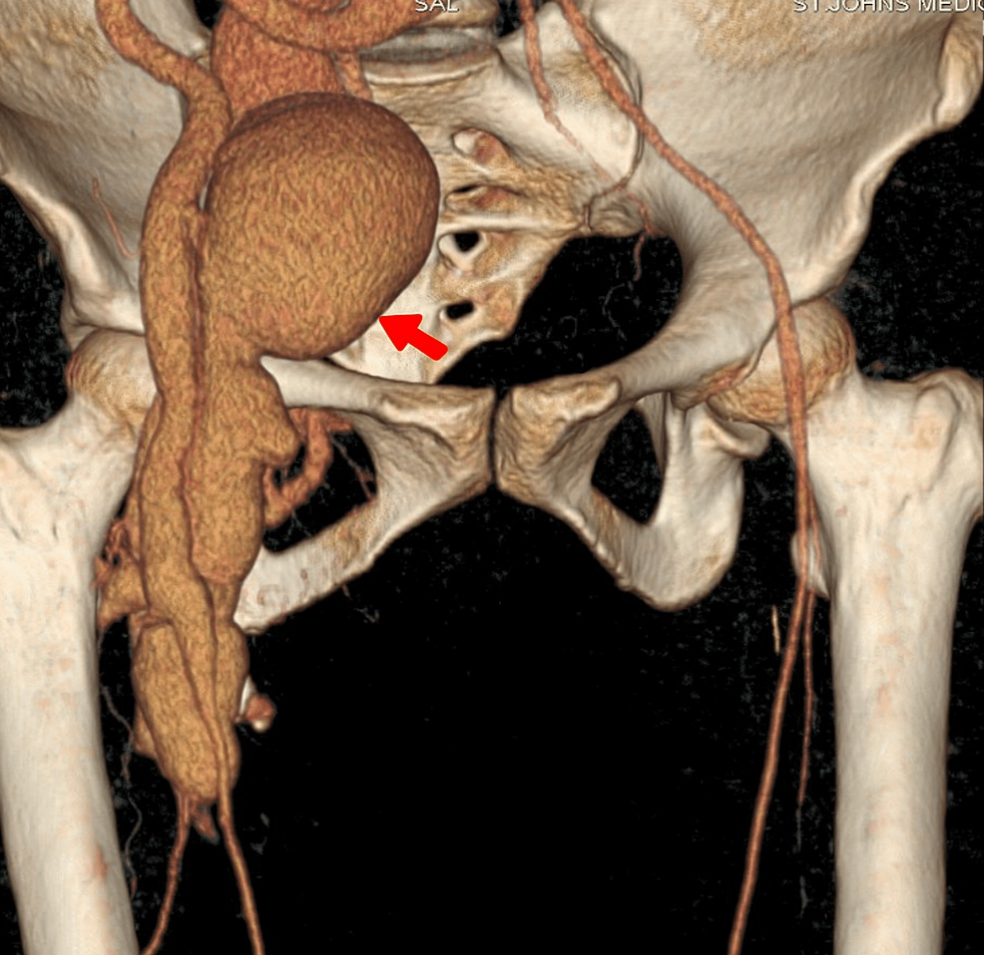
Arteriovenous fistula (indicated with an arrow) connection on CT of the abdomen and pelvis
